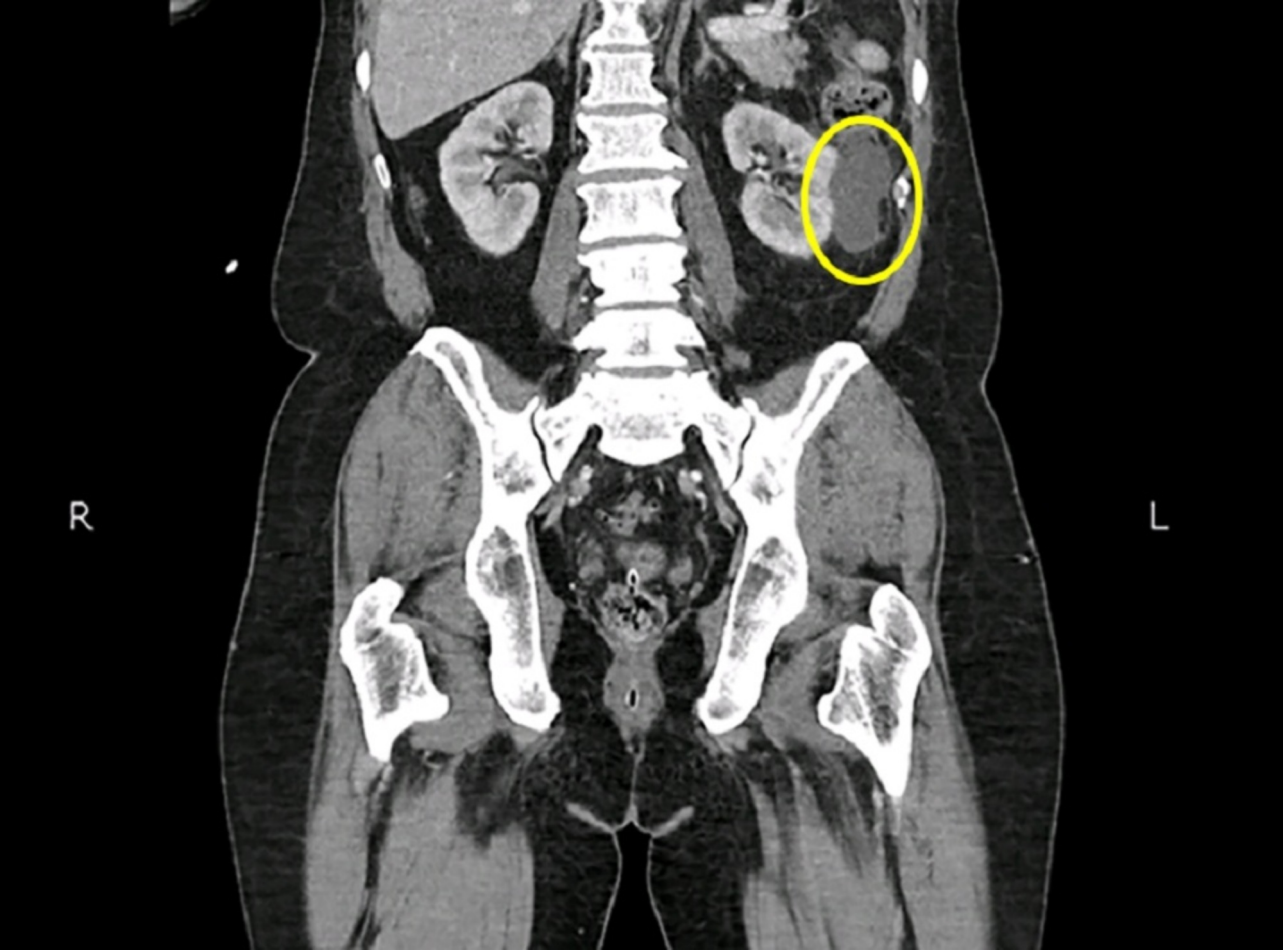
Computed tomography (CT) scan of the abdomen showing a heterogeneous lesion in the left kidney.
